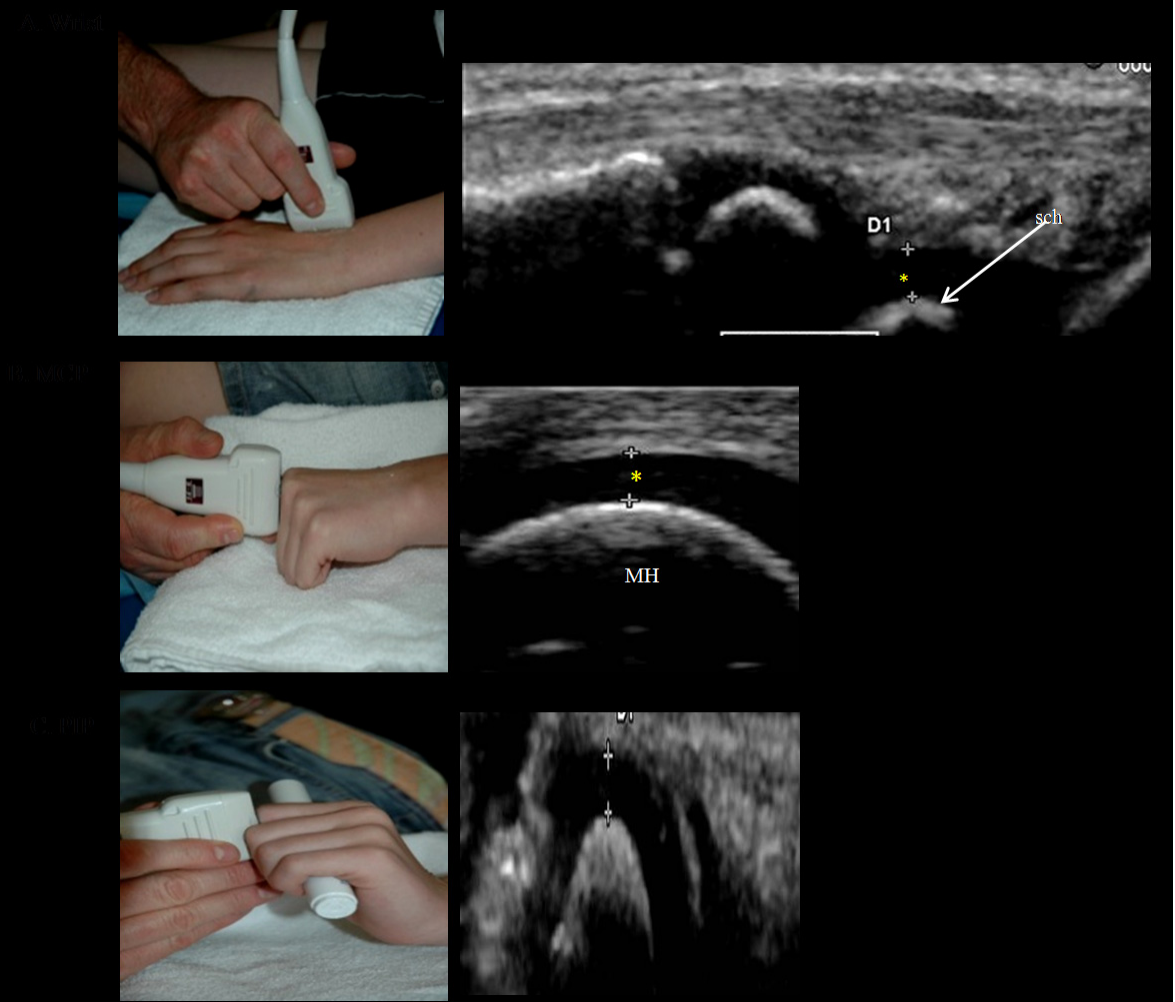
Ultrasonographic standard scans for cartilage thickness measurements in the wrist, metacarpophalangeal (MCP) and proximal interphalangeal (PIP). * = hyaline cartilage, R = radius, sch = os schaphoideum, MH = metacarpal head, DP = distal phalange.
